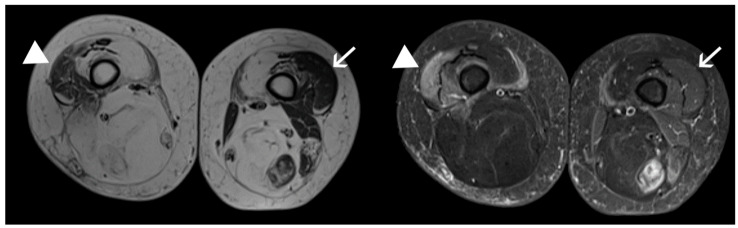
Muscles sampled by microdialyisis. STIR+ (arrowhead) and STIR- (arrow) vastus lateralis muscles sampled in the same patient. Axial STIR sequences of the thigh muscles are shown on the right-hand side, with corresponding T1-weighted images on the left.
